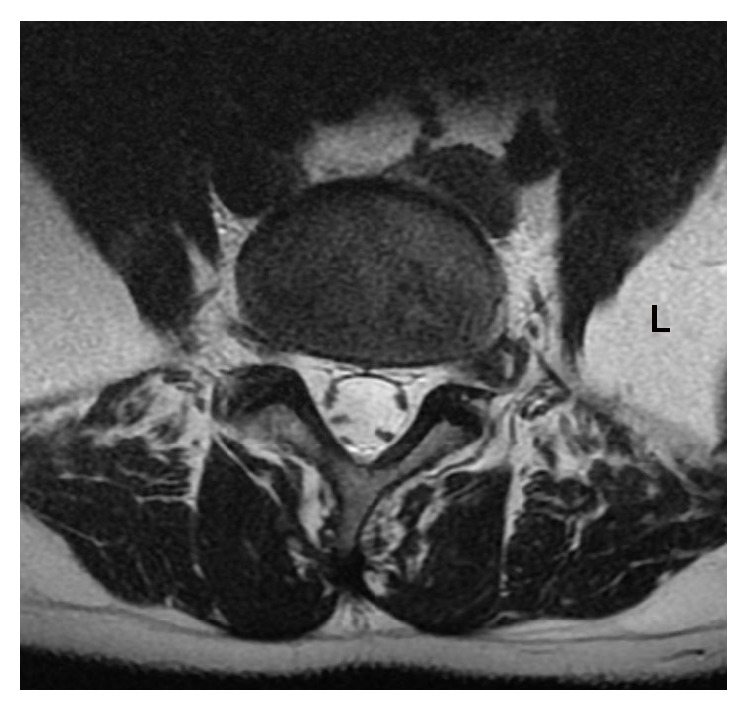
Axial T2-weighted MRI reveals extraforaminal ruptured disc on the left side (patient number 5). Therapy consists of removal of the fragmented disc segment without bone removal or discectomy. Navigation allows for a safe transmuscular approach.
